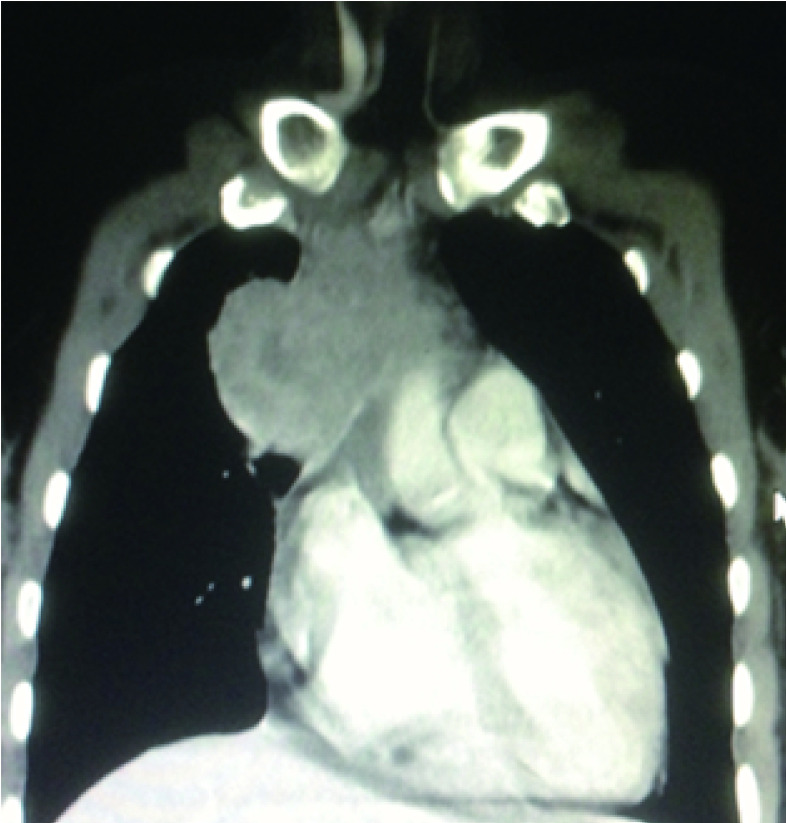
恶性胸腺瘤侵犯上腔静脉的CT征象 CT images malignancy-related superior vena cava syndrome.CT: computed tomography.
